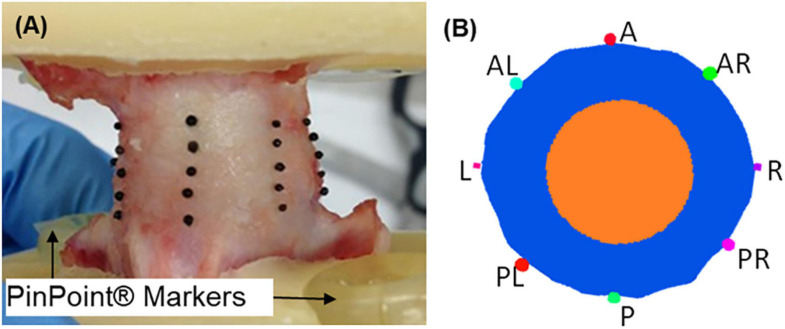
(A) Osteodisc prepared for mechanical testing, with glass fiducial markers, CT- and MR-compatible PinPoint^®^ markers, and PMMA endcaps. (B) Transverse plane slice through the intervertebral disc showing segmentation of the annulus fibrosus (blue), nucleus pulpous (orange) and fiducial glass beads, with classification of the eight zones of the disc (A, anterior; P, posterior; L, left; R, right).
